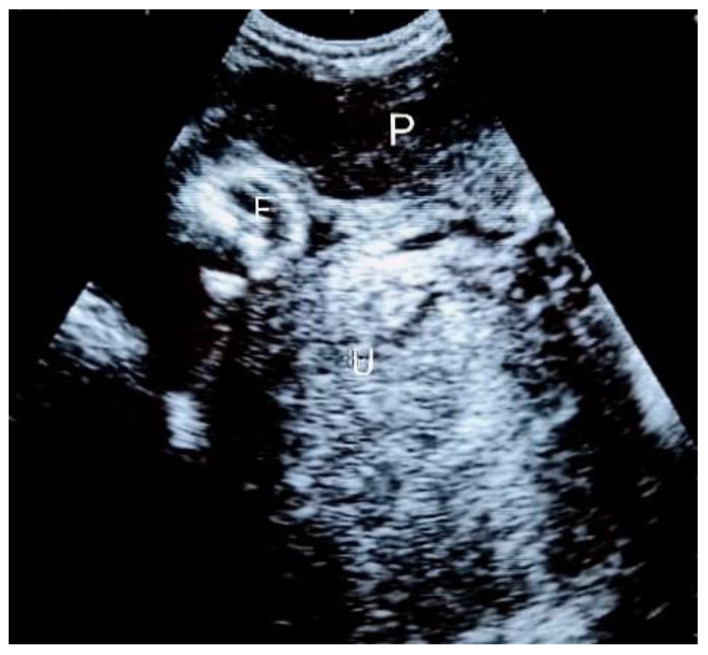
Third trimester abdominal ultrasound scan (left-lateral view) showing the fetal limbs, placenta, amniotic fluid, and the empty uterus. P = placenta; F = fetal limbs; U = uterus.
